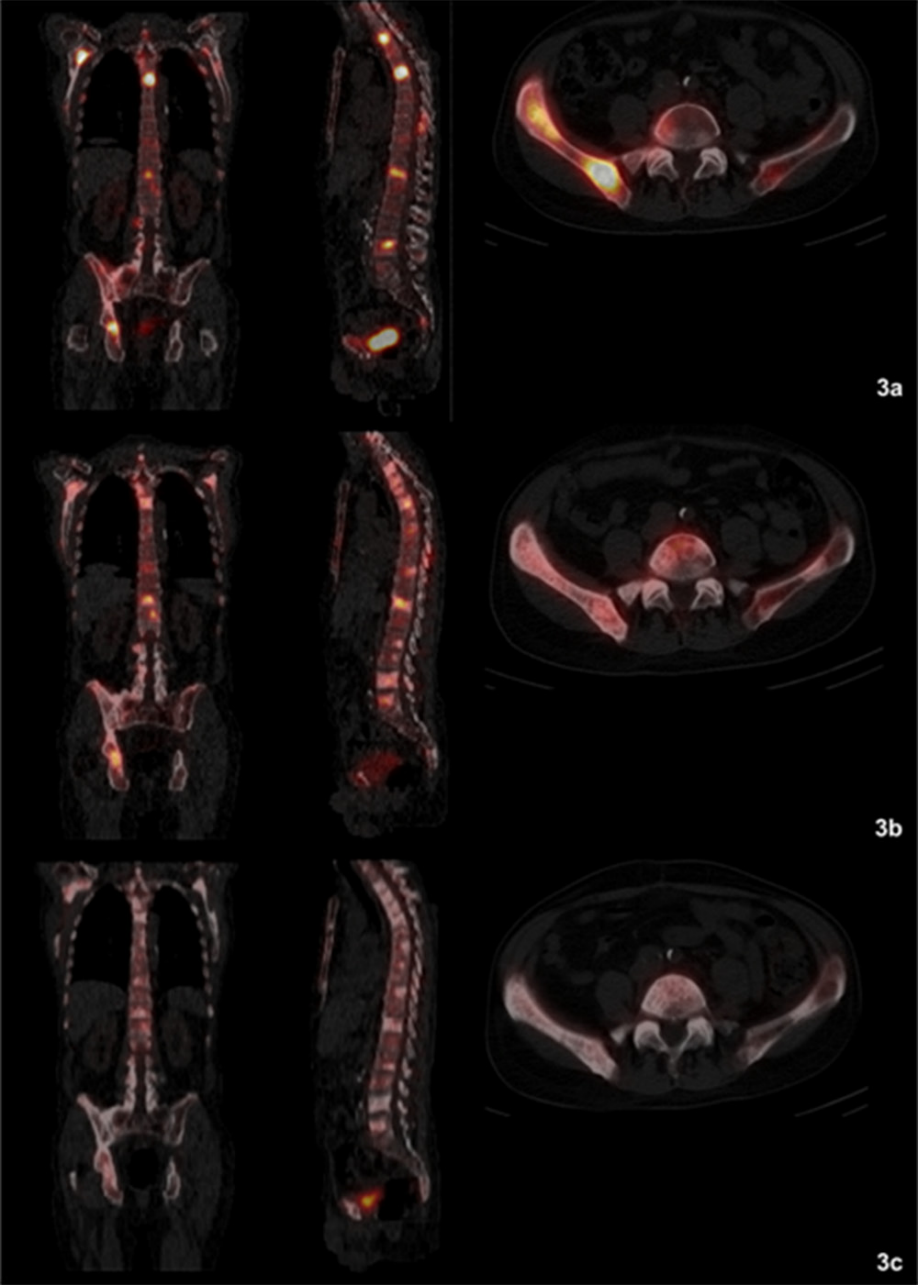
(a-c) Fused Single Photon Emission Computed Tomography (SPECT)/CT images clearly depicting a decreasing radionuclide uptake in the bone metastases (patient 4). (a) (top row): SPECT/CT before start of therapy (before cycle 1): Evidence of disseminated bone metastasis with high radionuclide uptake. (b) (middle row): SPECT/CT during therapy (between cycles 3 and 4): Decreasing uptake in bone metastases. (c) (bottom row): SPECT/CT at follow-up visit: Significantly decreasing and missing radionuclide uptake in bone metastases compared to baseline images. No evidence of new bone lesions.
